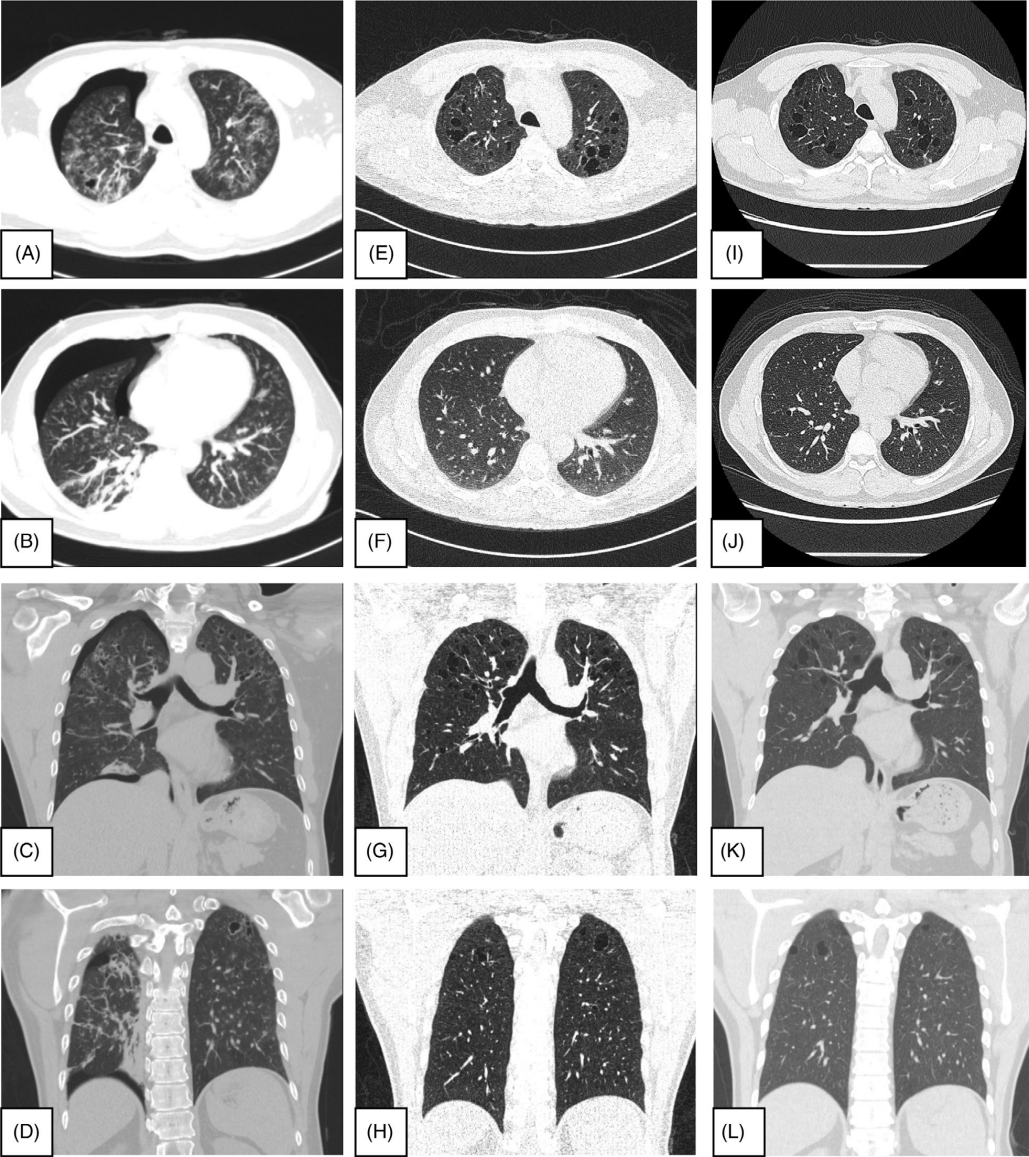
(A–D) Event initial episode. (E–H) Event after 3 months of smoking cessation. (I–L) Event after 14 months of tobacco cessation. Image examination by lung window of chest computed tomography via transverse and coronal view demonstrates bilateral lung, bizarre‐shaped and cavitary nodules, peripheral bronchioles tree in buds, various and numerous irregular air‐density parenchyma cysts, wall thickening improving without progression, decreased in size and numbers. Infiltration, consolidation, ground‐glass opacities and wall thickening of bronchioles became thinner and vanished
